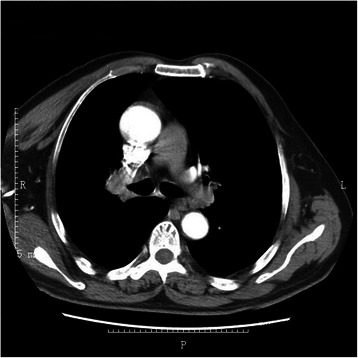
Chest computed tomography showing a tumor located around bronchus of the right upper lobe
